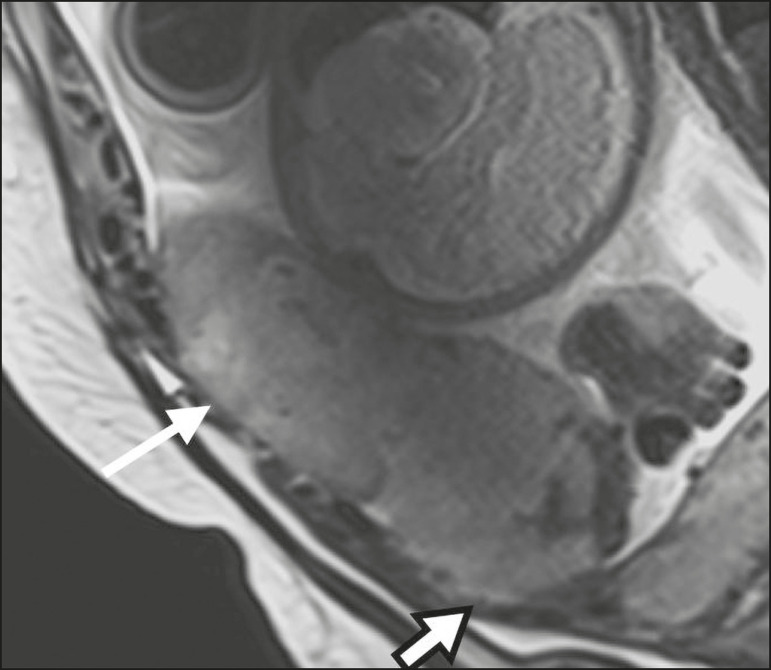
Sagittal T2-weighted MRI sequence showing a normal thin layer with low signal intensity between the myometrium and the placenta (white arrow) and an irregular placental-myometrial interface (outlined arrow).
